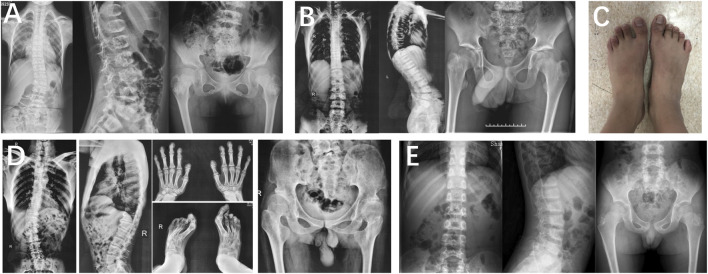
Photographs and radiographs of families 1 and 2 with TRPV4-related skeletal dysplasia. (A) Radiographs of the spine and pelvis of proband 1 (Ⅱ-1) in family 1. Mild scoliosis, ovoid vertebral bodies, and rough edges of the vertebral bodies were significant in the spine. Disorganization of metaphysis was in the pelvis. (B,C) Radiographs of the spine and pelvis (B), and photographs of feet (C) of proband 2 (Ⅲ-1) in family 2. (B) Rough edges of the vertebral bodies and lumbar lordosis were visible in the spine. Disorganization of the metaphysis and shortening of the femoral neck were in the pelvis. (C) Fourth metatarsal dysplasia was in the left foot. (D) Radiographs of hands, spine, pelvis, and feet of proband 2’s father (Ⅱ-1). Mild contracture of the distal joints was shown in the hands. Scoliosis and depression of the anterior edge of vertebral bodies were displayed in the spine. Flattening of the acetabular roof, shortening of the femoral neck, and stenosis of joint spaces were in the pelvis. Hallux valgus deformity was seen in feet. (E) Radiographs of the spine and pelvis of proband 2’s brother (Ⅲ-2). Irregular shape of the vertebral bodies in the spine. Radiographs of the pelvis were normal.
